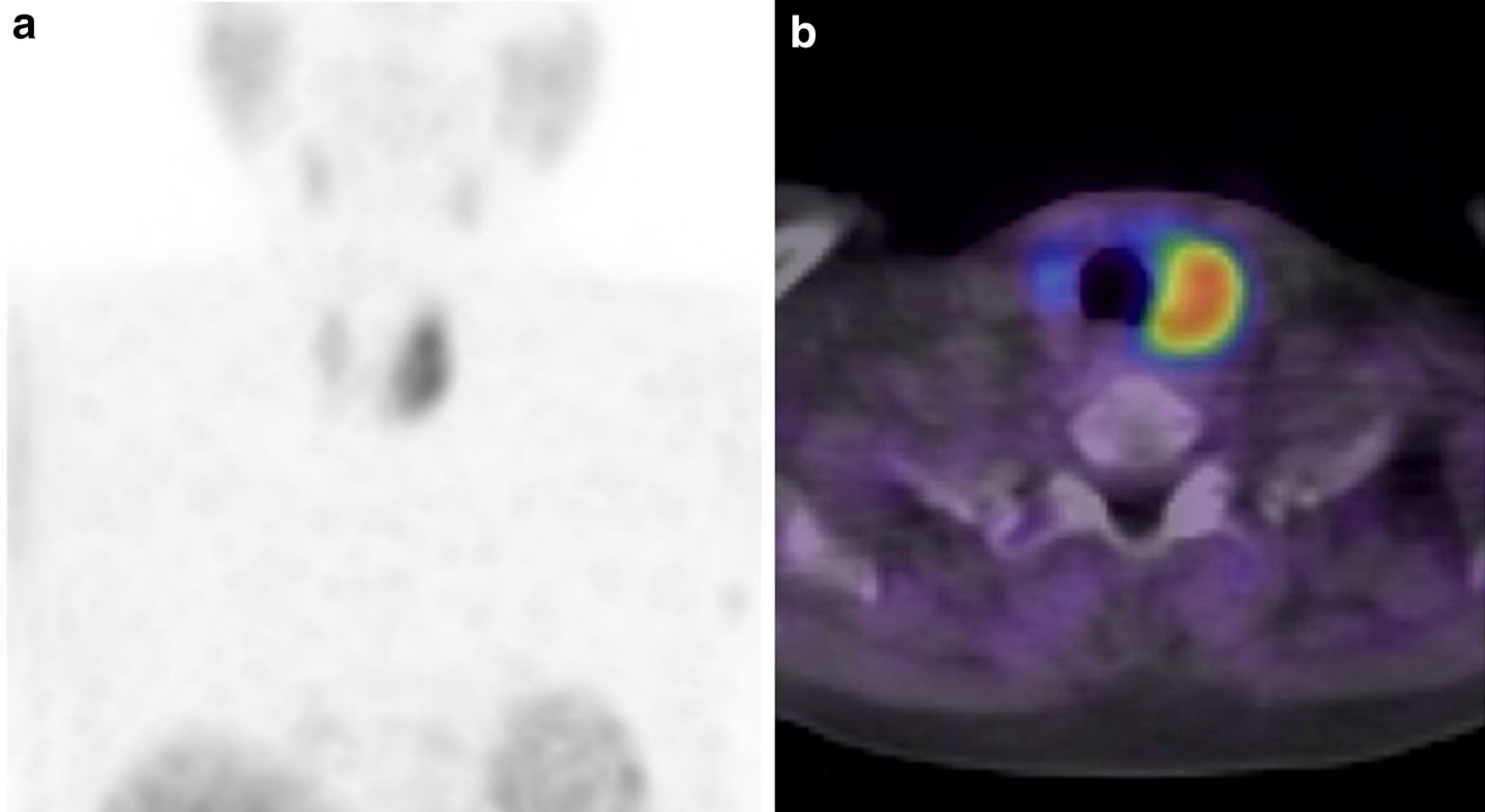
Case 1. a Delayed image of 99mTc-MIBI (99m-technetium-ethoxysobutylisonitrile) scintigraphy showing abnormal uptake on the left side of the parathyroid area, b SPECT-CT showing abnormal uptake in the same location
